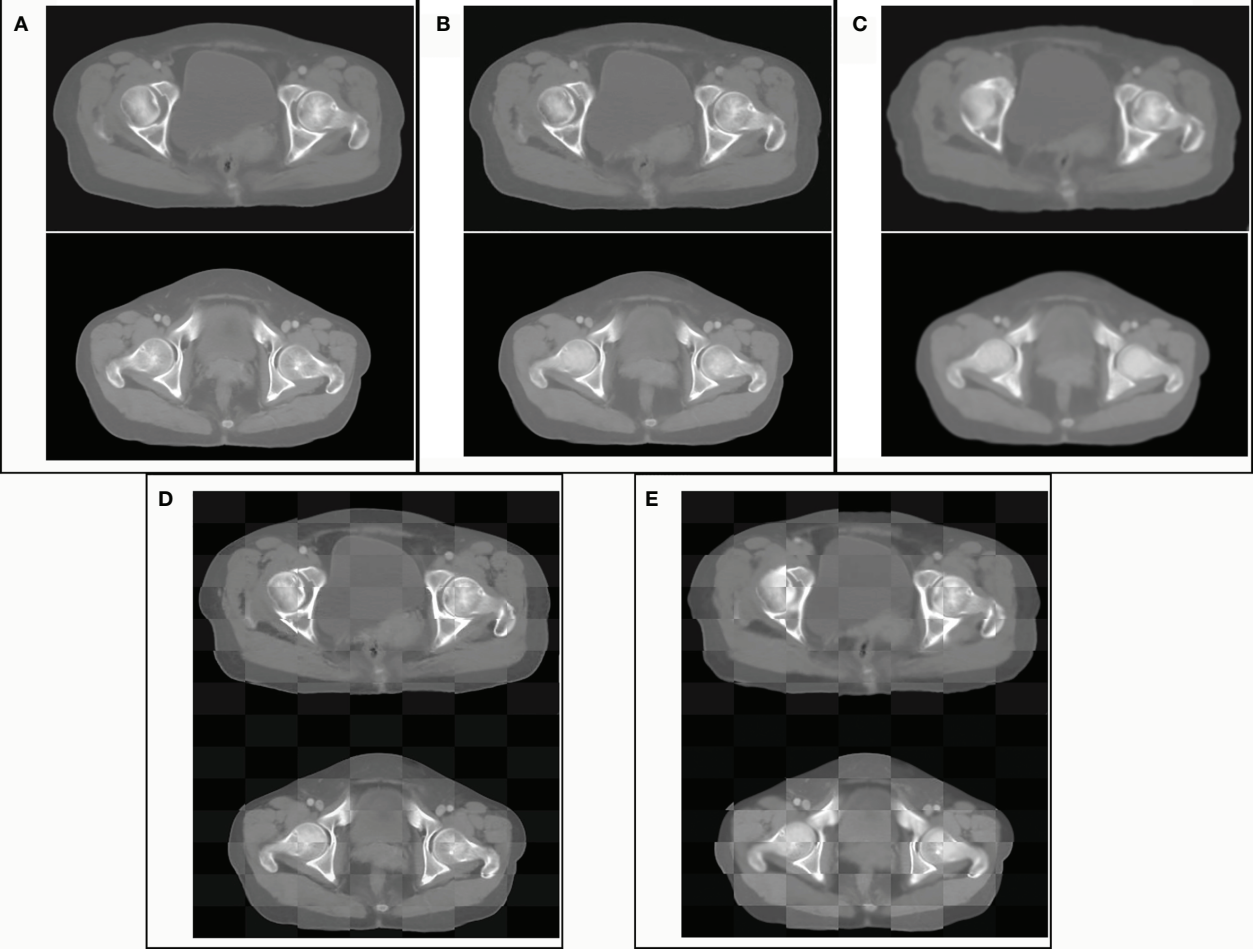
Results of pseudo-CT images obtained by adding gradient loss function and not adding it in CycleGAN. (A) CT_gt._
